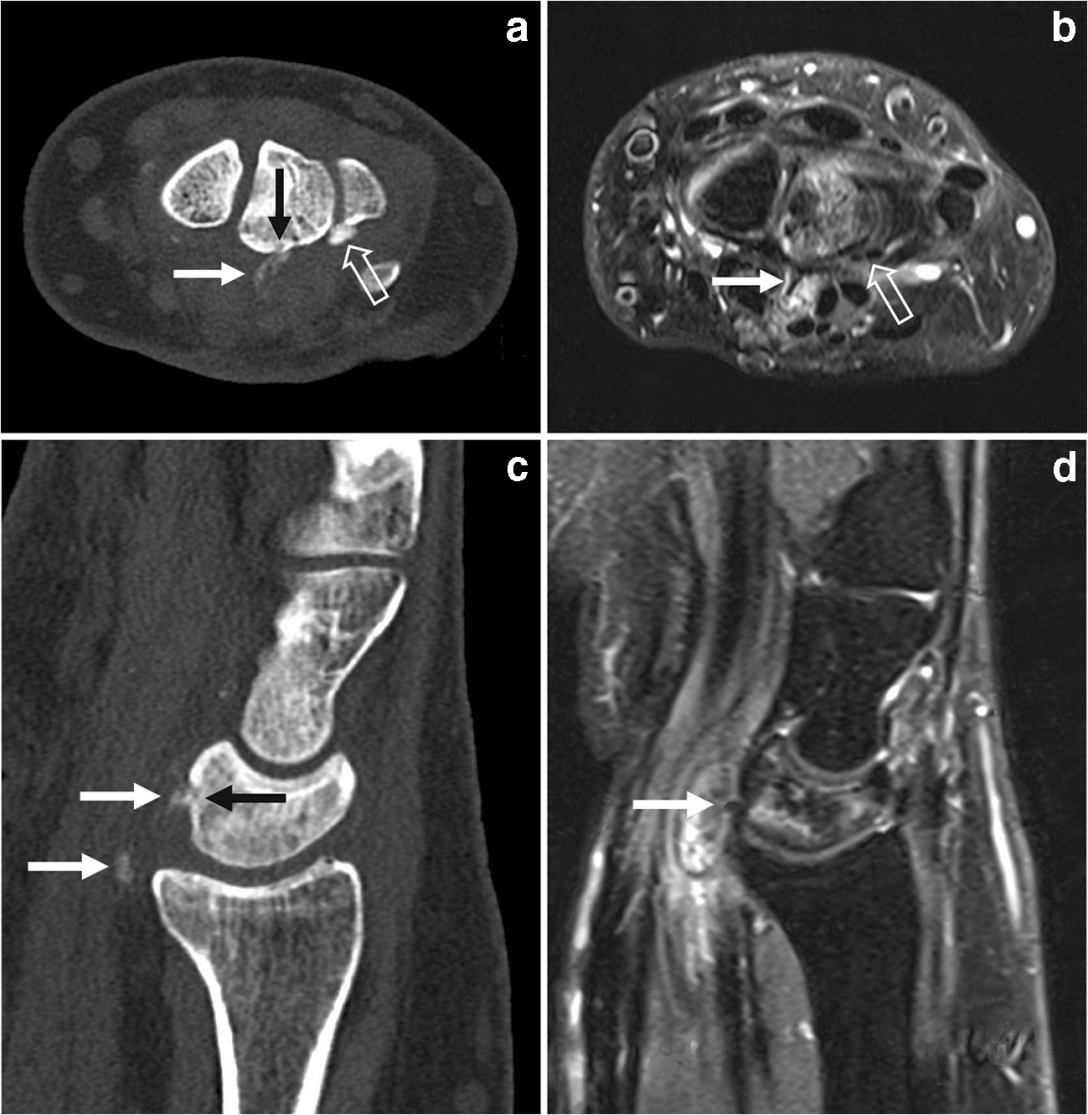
Transaxial and sagittal CT and MR images. a CT image with two calcified structures of different density. The tail-like, amorphous calcified depot (white arrow), consistent with already liquified HA shows intraosseous migration into the lunate bone. A small erosion is evident (black arrow). A dense, smooth-edged calcification depot located at the lunotriquetral junction is concordant with a calcific HA stage (open arrow). b In fat-saturated proton density–weighted MRI, both calcifications (white and open arrows) appear hypointense. They are surrounded by soft tissue oedema. An inhomogeneous bone marrow oedema is evident in the lunate bone. c In the sagittal CT image, loosened calcifications are present in the depth of the carpal tunnel (white arrows). There is an erosion at the cortex at the anterior horn of the lunate bone (black arrow). The small erosive defect is filled with calcified depots. d In sagittal MRI (fat-saturated proton density–weighted), the calcifications are just visible as hypointense spots, one of them located directly at the anterior horn of the lunate bone (white arrow). In the carpal tunnel, there is a space-occupying oedema of soft tissues with volar displacement of the deep flexor tendons. The bone marrow oedema of the lunate bone is patchy and inhomogeneous
